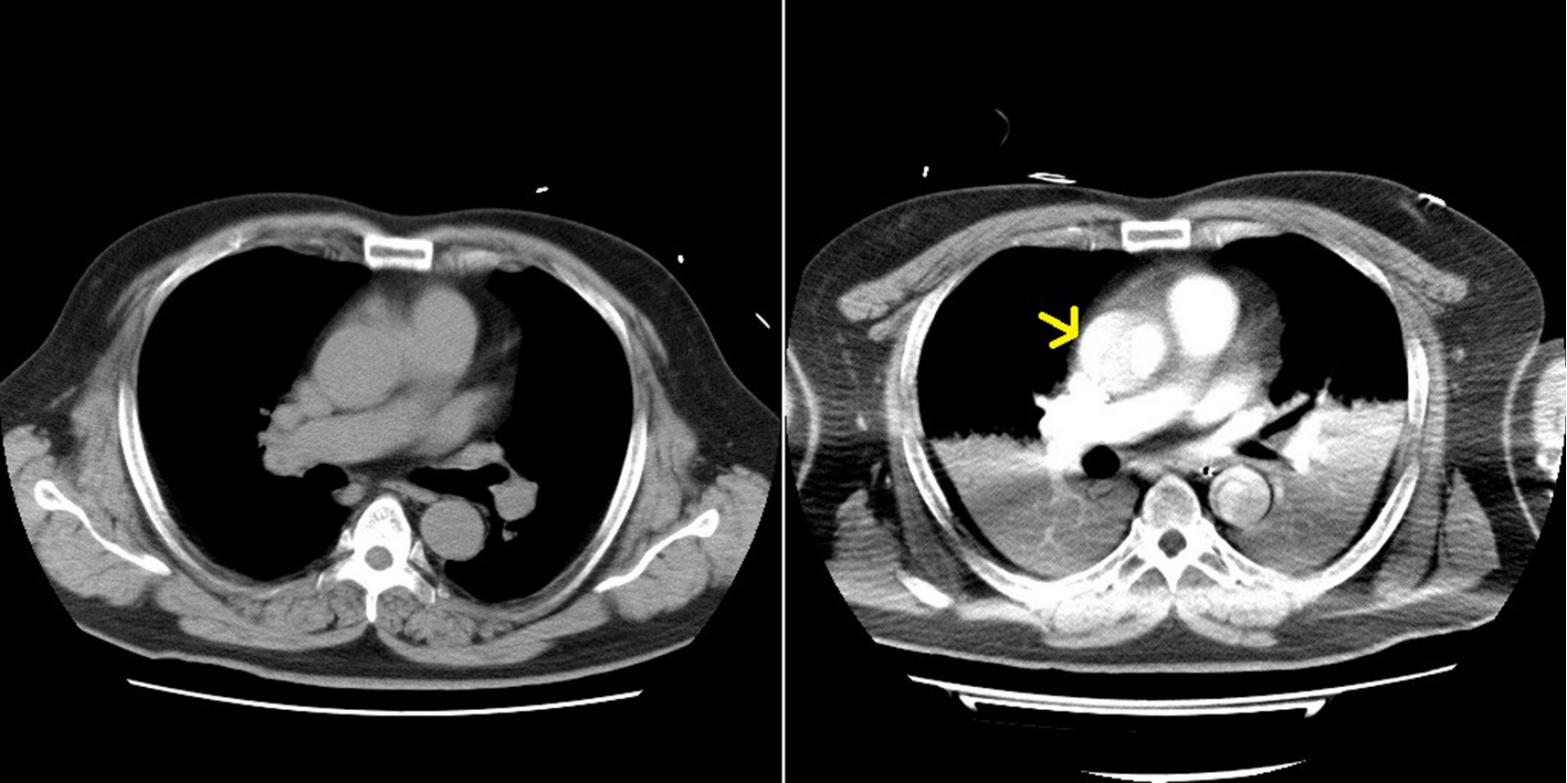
Computed tomography imaging. Plain computed tomography (CT) imaging demonstrated no definite AAD (on left). Contrast‐enhanced CT imaging after PCI demonstrated Stanford type‐A AAD (on right; a yellow arrow points out the false lumen of the ascending aorta). AAD, acute aortic dissection; PCI, percutaneous coronary intervention.
